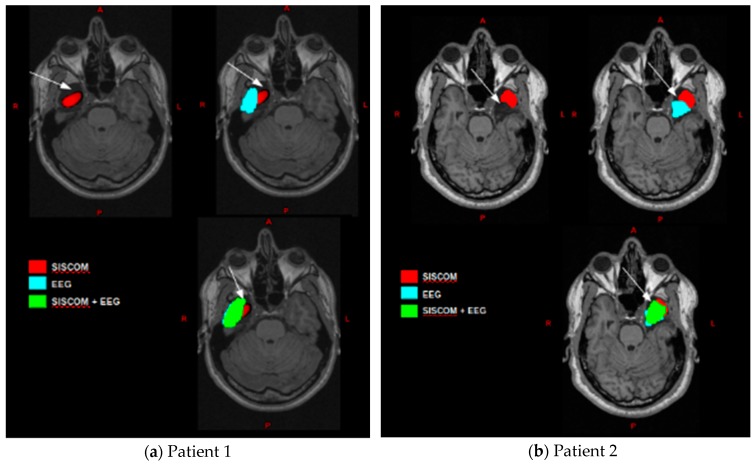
Co-registration of post-operative T1 with EZ images obtained by: subtraction of ictal and interictal SPECT co-registered with MRI (SISCOM) (in red), ictal EEG source imaging (ESI) (blue) and ictal ESI using SISCOM as a prior (green); (a) for patient 1; and (b) for patient 2. A white arrow indicates resection zone.
